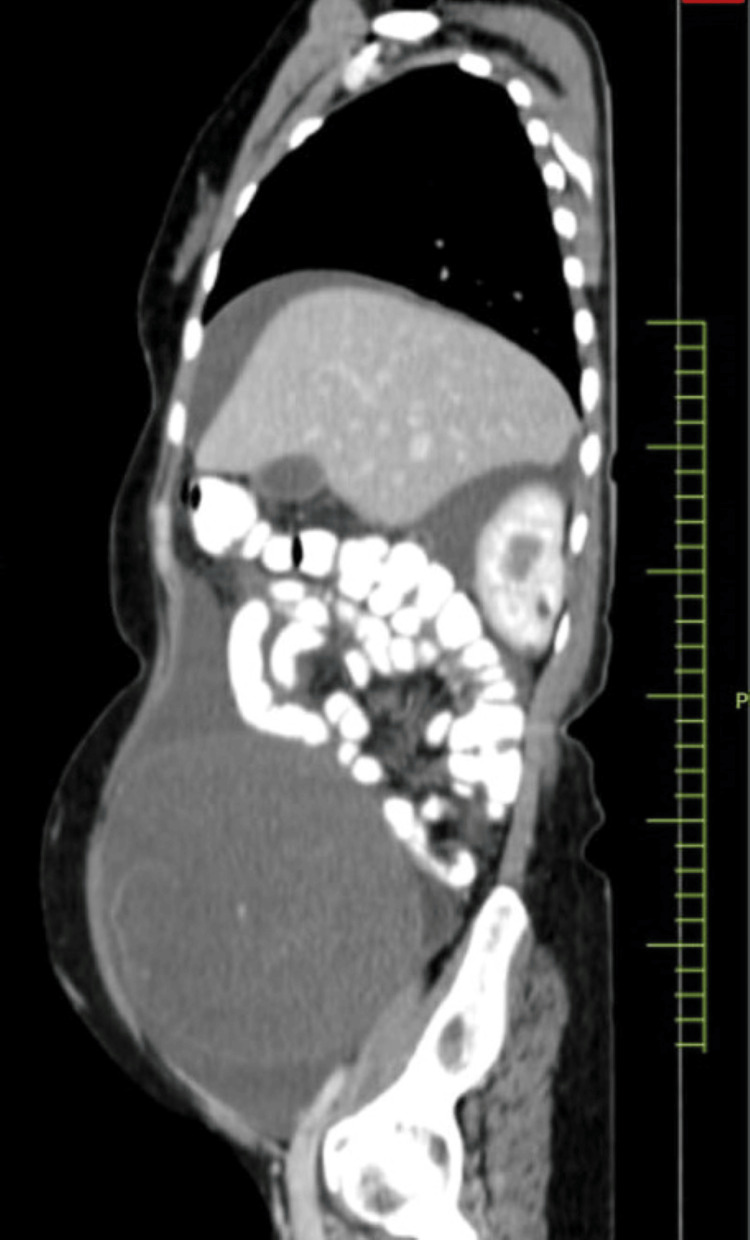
CT image shows a large, well-defined, solid cystic lesion in the pelvis in the midline, extending up to the level of the umbilicus and measuring 185 × 141 × 201 mm
